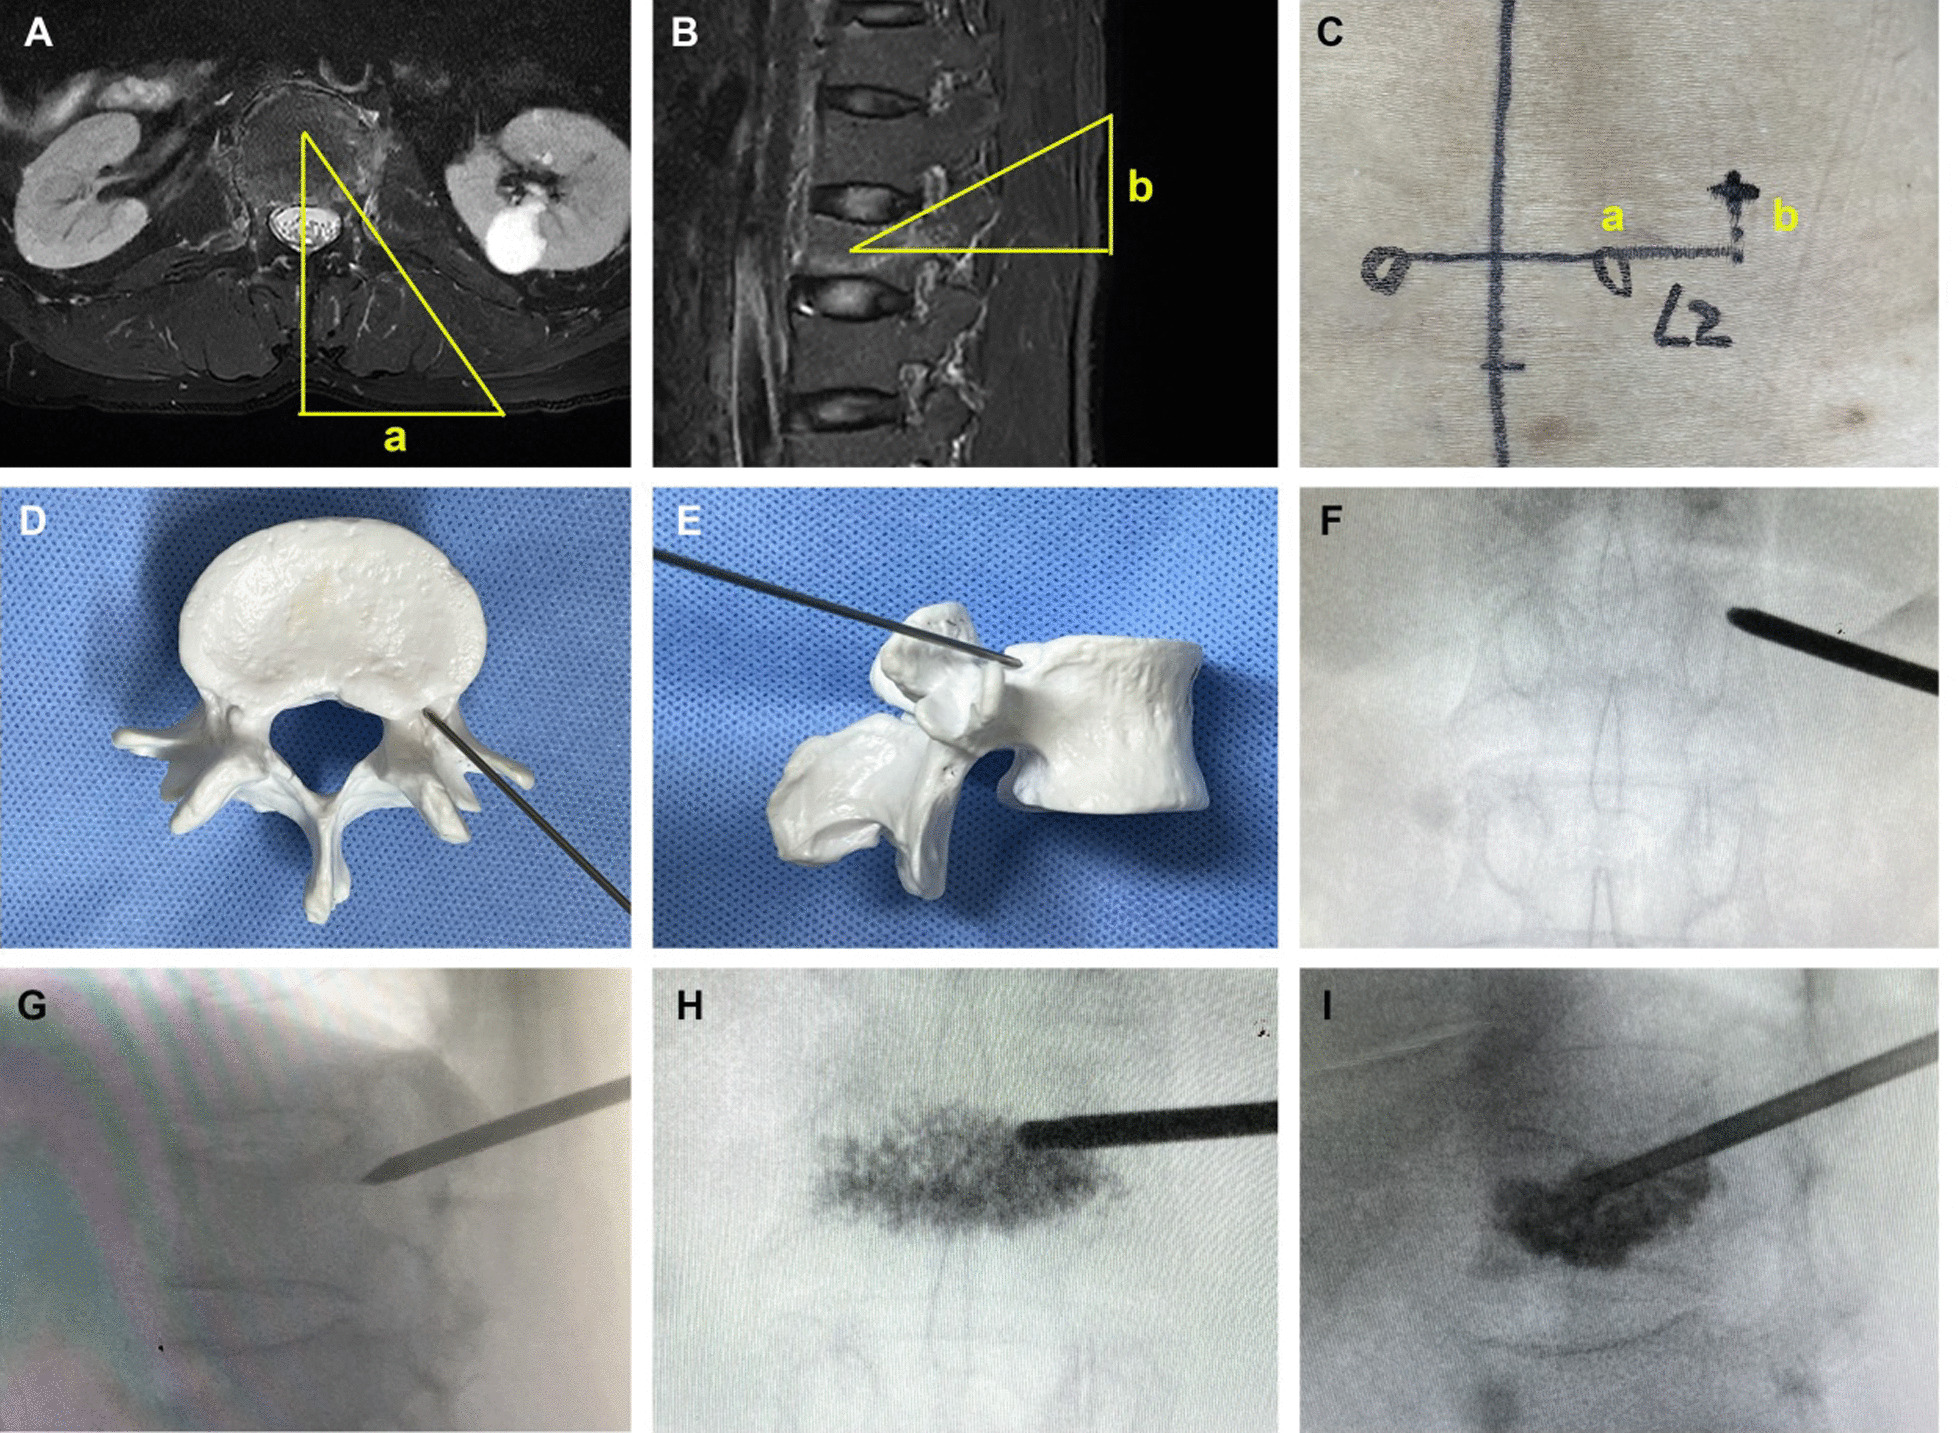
Kyphoplasty by unilaterally extrapedicular approach. A On the axial MRI film, a indicates the distance between the spinous process and the puncture site. B On the sagittal MRI film, b indicates the distance between the puncture site and the line crossing pedicles. C The puncture site on skin based on preoperative MRI measurements. D, E the entry site is located on the superolateral periosteum relative to the pedicle. F, G, H, I the surgical procedure of kyphoplasty by the unilaterally extrapedicular approach
